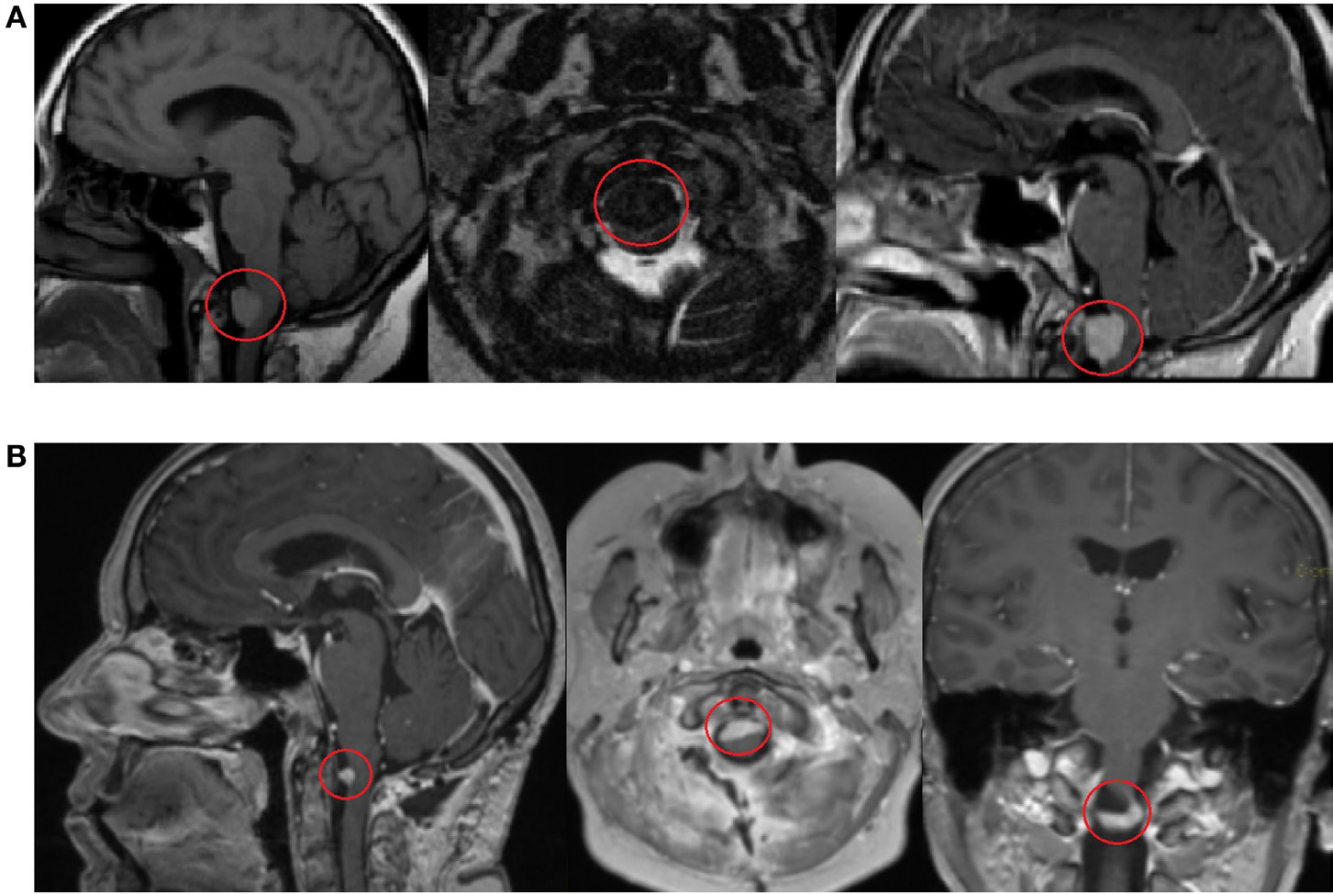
Contrast-enhanced head and brain magnetic resonance imaging (MRI) scan shows a formation in the craniospinal junction before surgery on 17 March 2014 (A). Contrast-enhanced MRI scan 3 months after surgery shows a minimal, residual caudal part of the tumor on 5 June 2014 (B).
